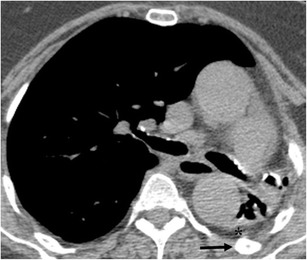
A 76-year-old female with TB history in infancy presenting with dry cough for 1 month. Non-enhanced CT shows total unilateral left lung destruction with bronchiectasis. Upper ipsilateral ribs are hypertrophic (black arrow) and extrapleural fat proliferation is also evident (asterisk)
